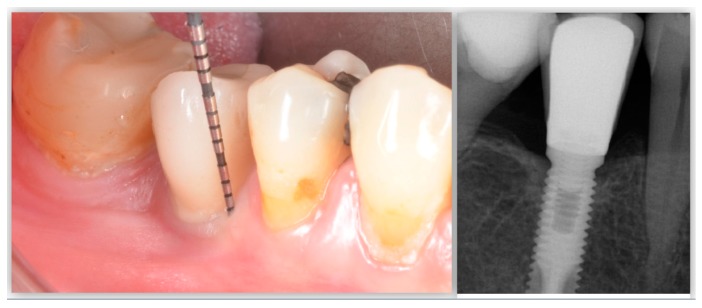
Representative case of an erratic maintenance complier with inadequate personal-administered oral hygiene presenting with healthy clinical and radiographic peri-implant conditions in the presence of 2 mm of keratinized and attached mucosa.
